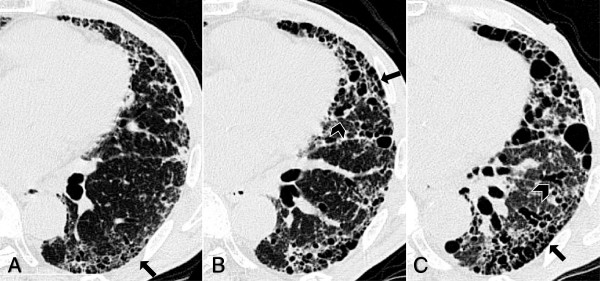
High-resolution CT findings of the patient with an adverse prognosis. The patient was a 78-year-old man with IPF. HRCT scan at the level of lower left lobe shows fibrosing changes from the baseline (A) at six (B) and 12 months (C). HRCT images at diagnosis of IPF show subpleural predominant interstitial fibrosis, traction bronchiectasis (arrowheads) and honeycombing (arrows). The overall HRCT fibrosis score at the baseline, six and 12 months were 151.3, 162.5 and 185.8, respectively. This patient died 20 months after initial diagnosis.
